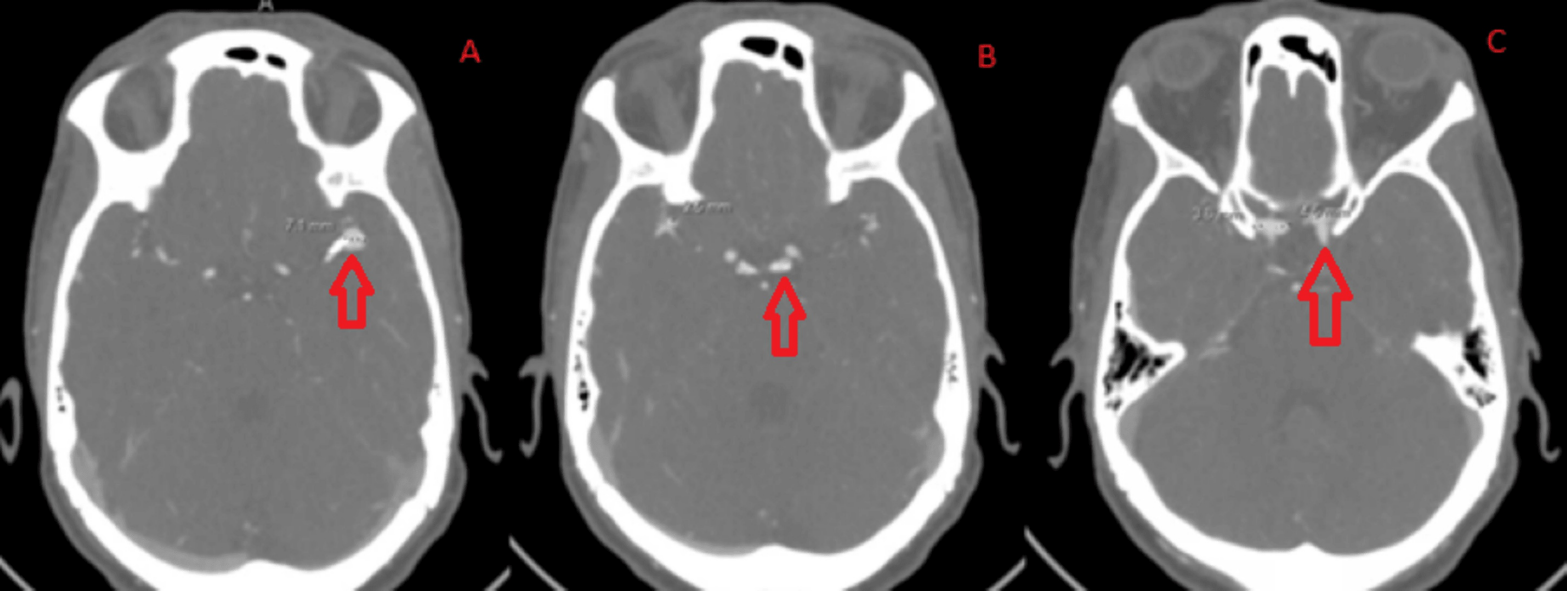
Follow-up computed tomography angiography two days after the procedure at high (A), middle (B), and deep (C) cross-sections.
